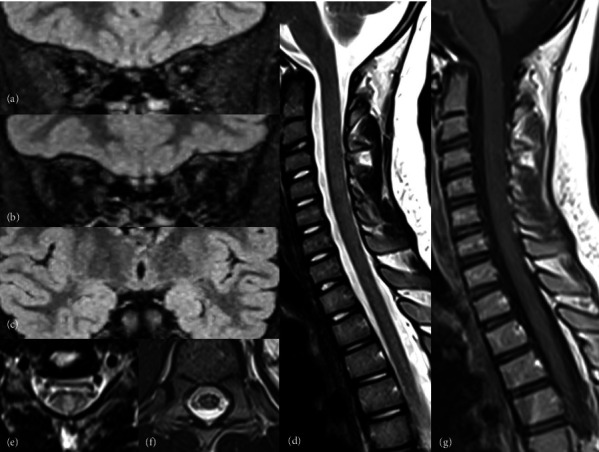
Control cerebrospinal and orbital MRI after biotin supplementation. (a–c) Coronal fluid–attenuated inversion recovery weighted images showing a normal signal on the optic nerves and mamillary bodies. Sagittal (d) and axial (e, f) T2 weighted images on the spinal cord. Persistence of a mild hyperintense signal of the cervical posterior tract. (g) Sagittal T1 weighted image after gadolinium administration. Disappearance of the medullary enhancement.
